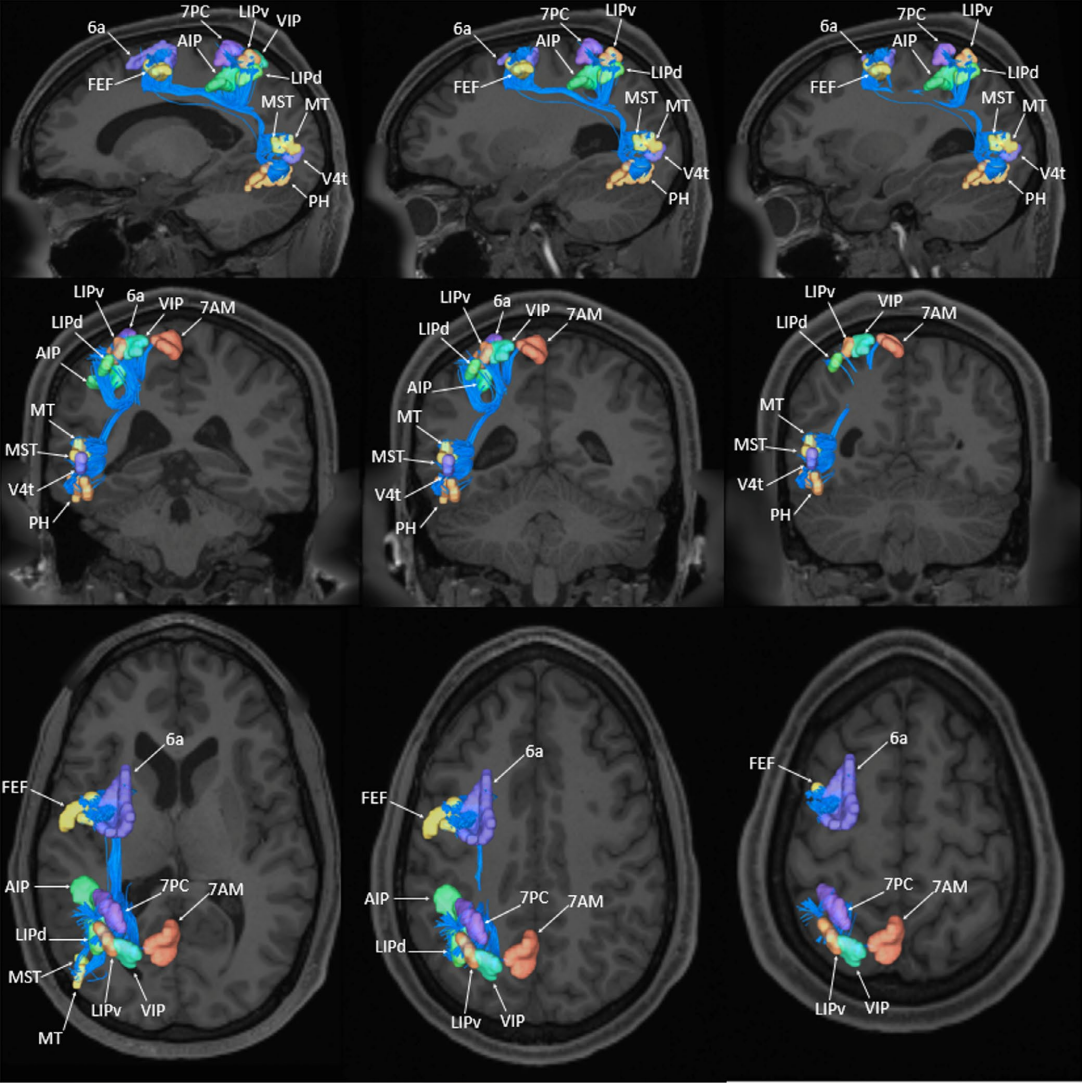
Tractographic model of the dorsal attention network (DAN) as shown on T1‐weighted magnetic resonance images in the left cerebral hemisphere. TOP ROW: sagittal sections through the network demonstrate the extent of the superior longitudinal fasciculus (SLF) which projects between the frontal, parietal, and occipital regions of the DAN. MIDDLE ROW: coronal sections highlight the parieto‐occipital projections of the SLF within the DAN. BOTTOM ROW: axial sections highlight the fronto‐parietal projections of the SLF within the DAN
